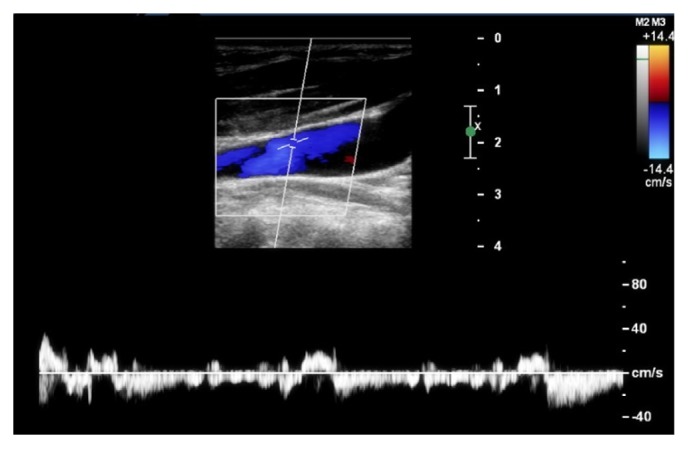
Retrograde flow detected in the Doppler spectrum spontaneously is considered jugular venous reflux (JVR).
